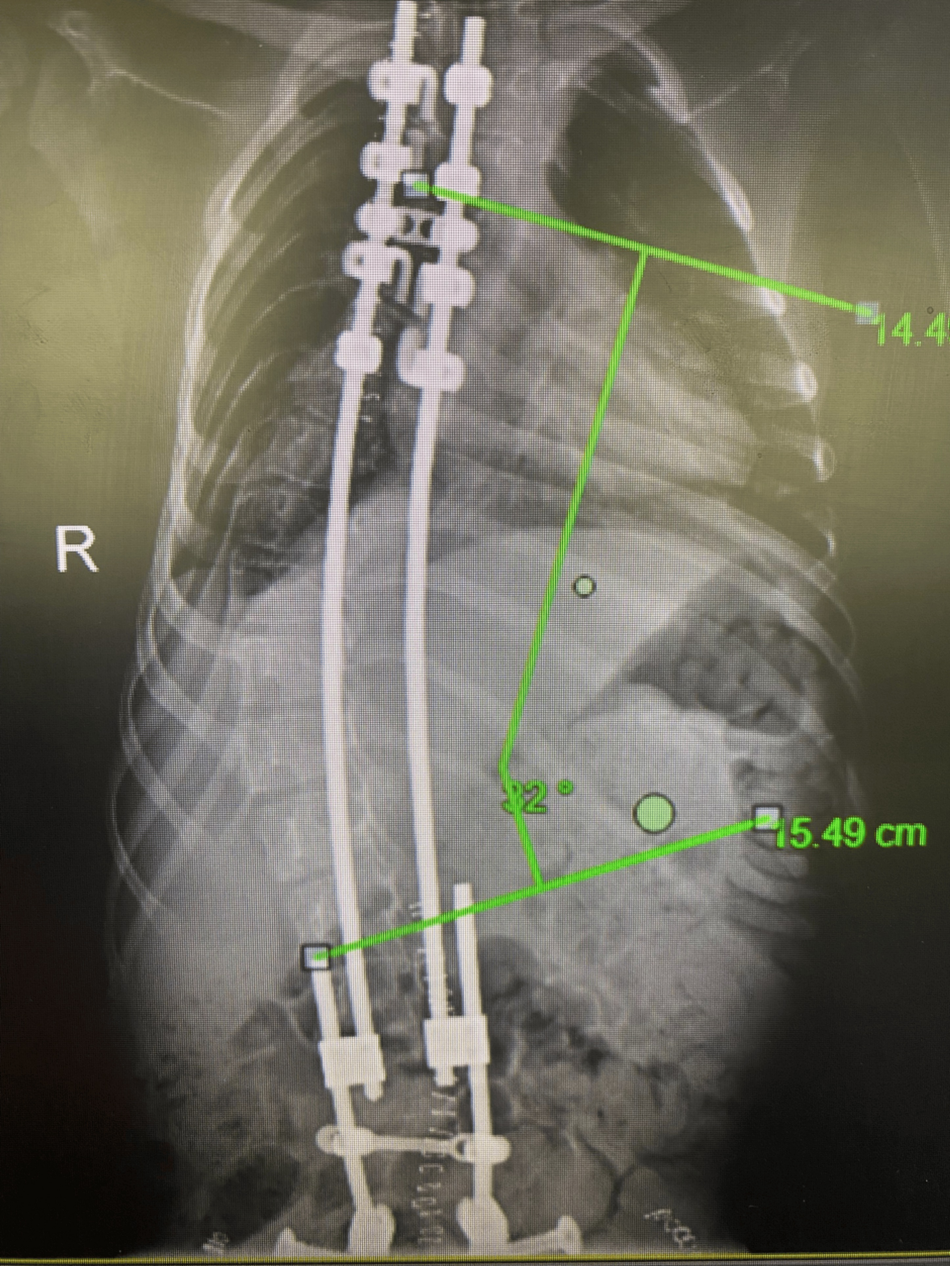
Postoperative correction of the Cobb angle on AP full-spine radiograph AP: Anteroposterior
